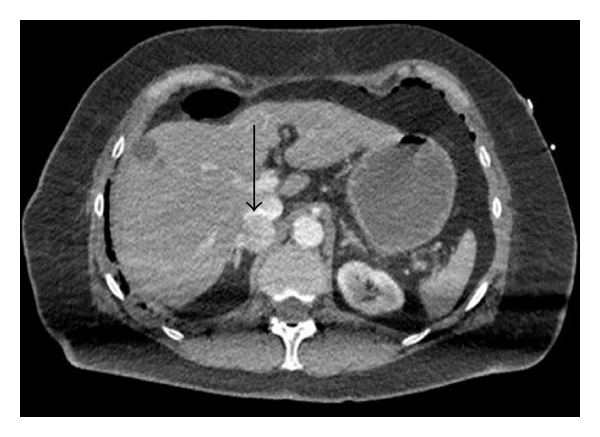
CT abdomen revealing a 22 mm nodule arising from the body of the right adrenal gland (arrow).
